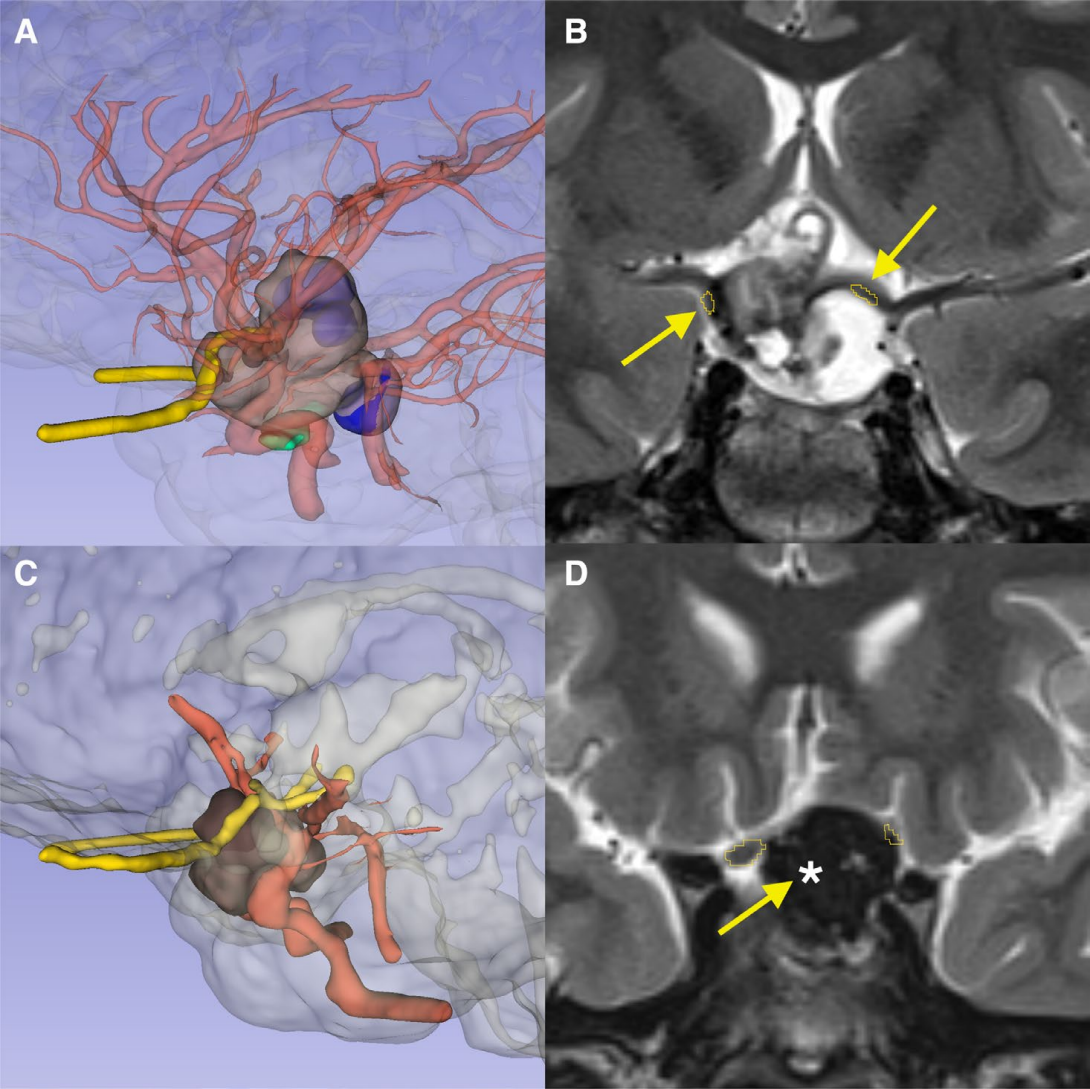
Preoperative coronal fat‐saturated T2‐weighted image (A) and corresponding 3D reconstruction (B) shows the craniopharyngioma compressing and displacing the hypothalamus, optic nerves (FIGURE arrow and outlined in yellow), and surrounding critical structures. Postoperative MRI (C) and 3D reconstruction (D) demonstrate near‐complete restoration of anatomy, with no detectable tumor remnants. The surgical cavity (yellow arrow and asterisk) remains in close proximity to the optic nerves and hypothalamus.
